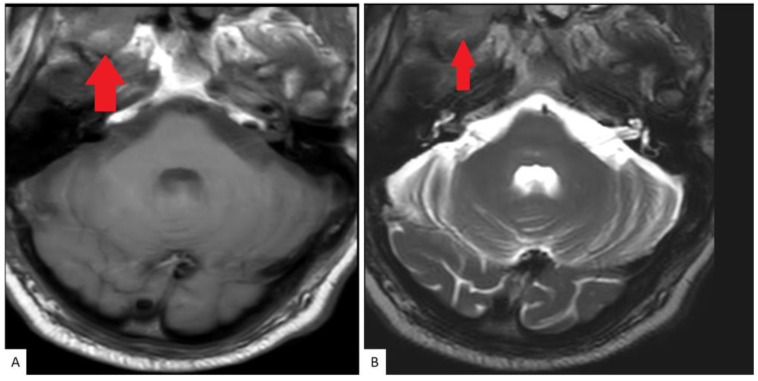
MRI Cervical spine: Axial T1 (A) and T2 (B) weighted images as part of a cervical spine MRI demonstrate a soft tissue lesion centered in the right maxillary sinus involving the masticator space (red solid arrow).
